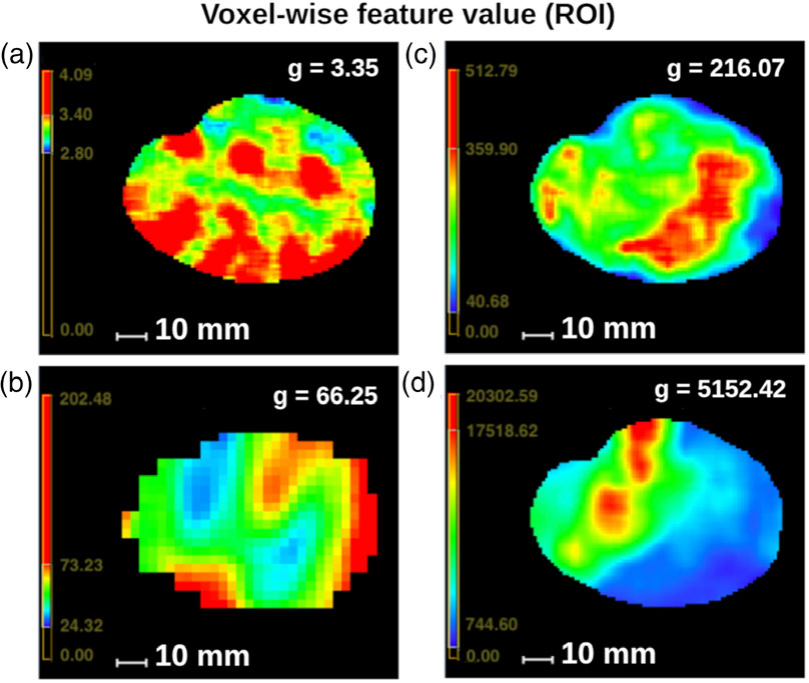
Example of engineered radiomic feature maps. As the models were trained by taking the mean values inside the region of interest as inputs, there was no need to resample all the feature maps on a common grid at this stage. The feature maps then had different spatial resolution (3 mm × 3mm × 3 mm for positron emission tomography (PET), 1 mm × 1 mm × 1 mm for computed tomography (CT) and magnetic resonance imaging (MRI)). (a) CT first‐order entropy, (b) PET gray‐level co‐occurrence matrix (GLCM) contrast, (c) T1 gray‐level dependence matrix (GLDM) gray‐level non‐uniformity (GLNU), and (d) fat‐suppressed T2 gray‐level run length matrix (GLRLM) long run high gray‐level emphasis (LRHGLE)
